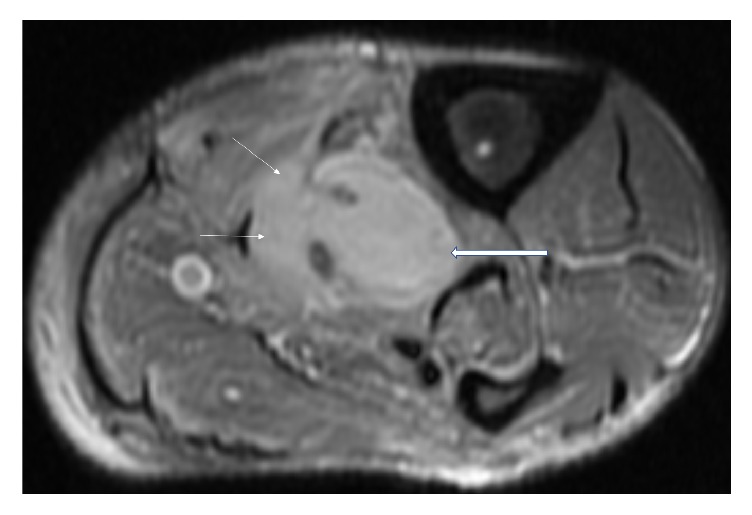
Axial, T2 weighted, fat saturated, STIR MR image showing loculated fluid signal within the tibialis posterior muscle (thick white arrows), suggestive of a collection. Also noted are diffuse T2 hyperintense signal changes in the surrounding musculature (thin white arrows), compatible with myositis.
